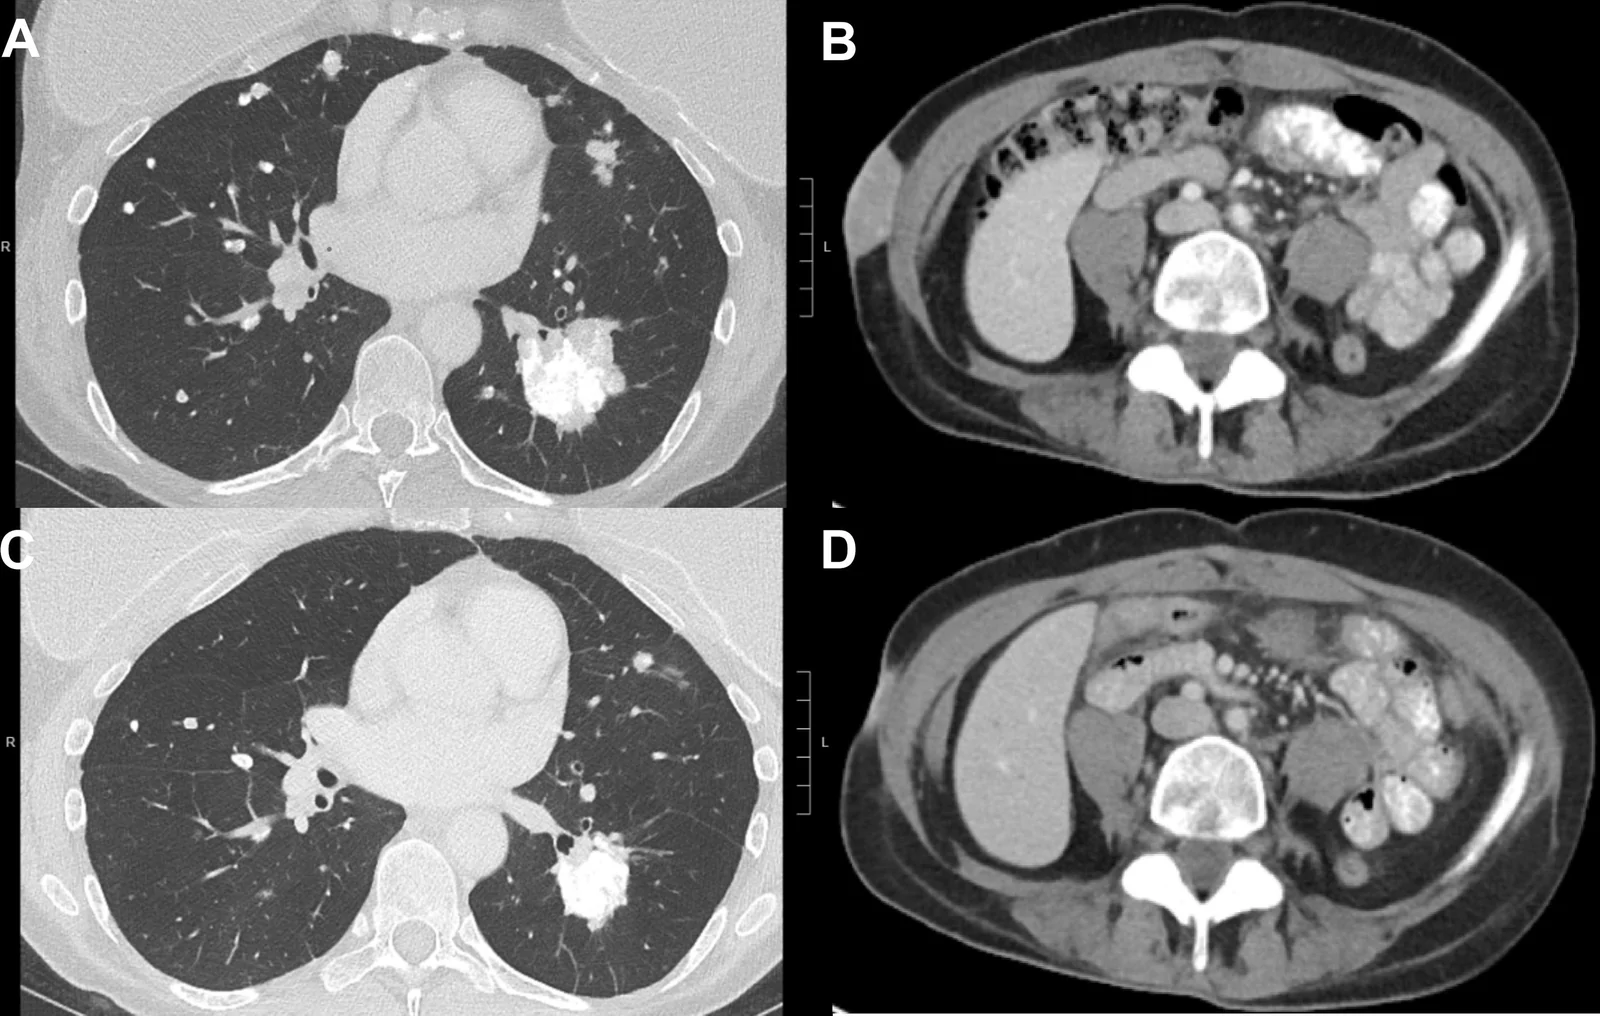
A-B: CT scans prior to initiation of dabrafenib monotherapy highlighting multiple pulmonary nodules (A) and a R flank soft tissue mass (B). C-D: CT scans obtained after 6 weeks of dabrafenib monotherapy demonstrating significantly reduced size of multiple pulmonary nodules (C) and R flank soft tissue mass (D).
